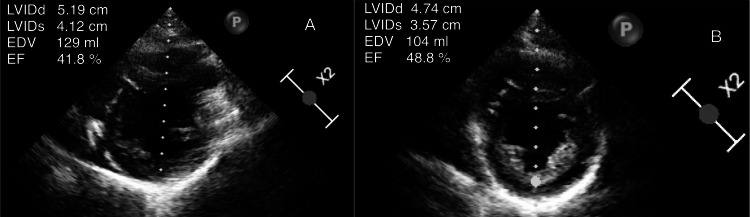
Parasternal short-axis view. (A) At diagnosis suggesting LVEF of 41.8%. (B) At the one-month follow-up with an improved LVEF of 48.8%. LVEF = left ventricular ejection fraction
